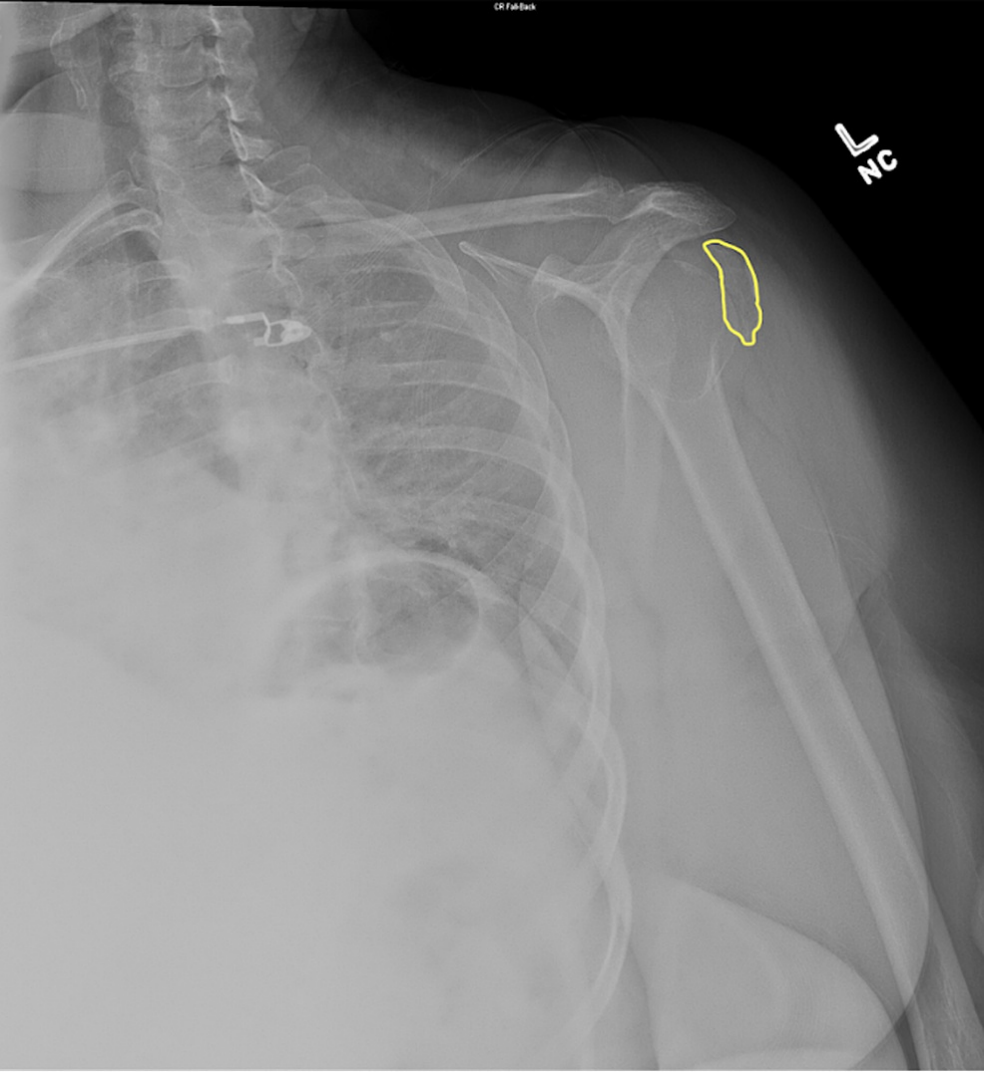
Patient’s shoulder radiograph post-reduction with an outline of the Hill-Sachs fracture.
